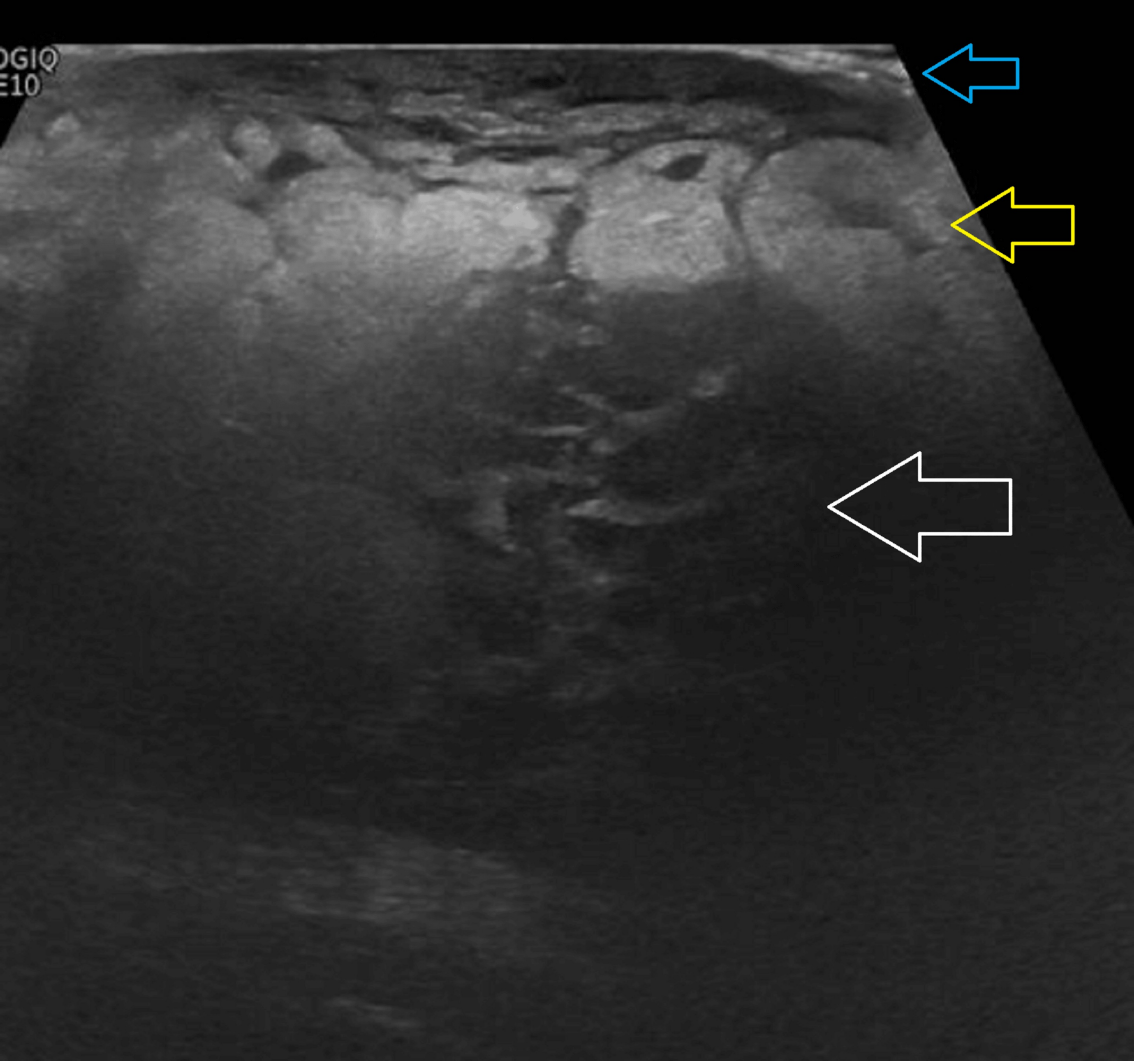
Ultrasound of the breast This image demonstrates increased skin thickness (blue arrow), underlying subcutaneous edema (yellow arrow), and diffuse infiltration of the breast tissue (white arrow), consistent with lymphoma involvement
